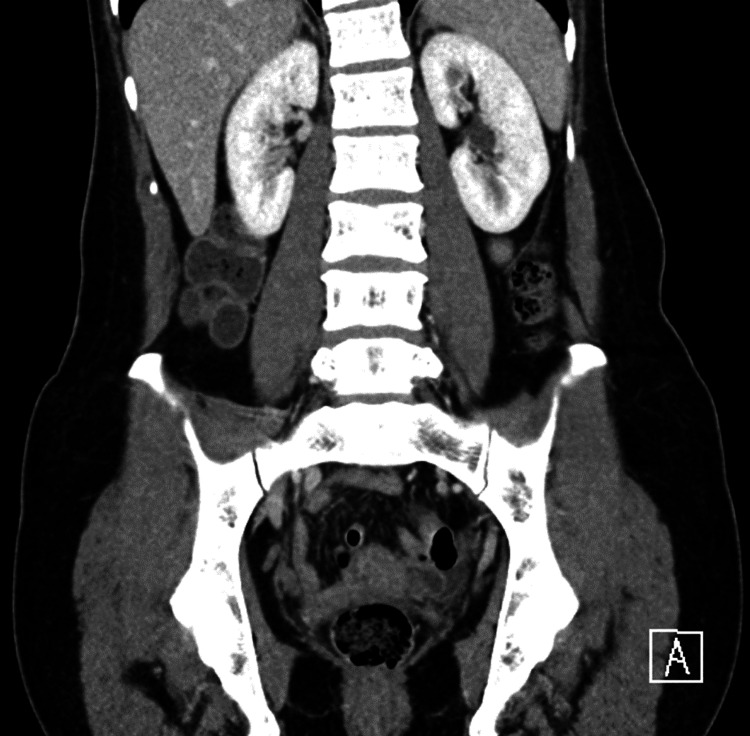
Coronal section of a contrasted computed tomography of the abdomen and pelvis, without acute findings.
